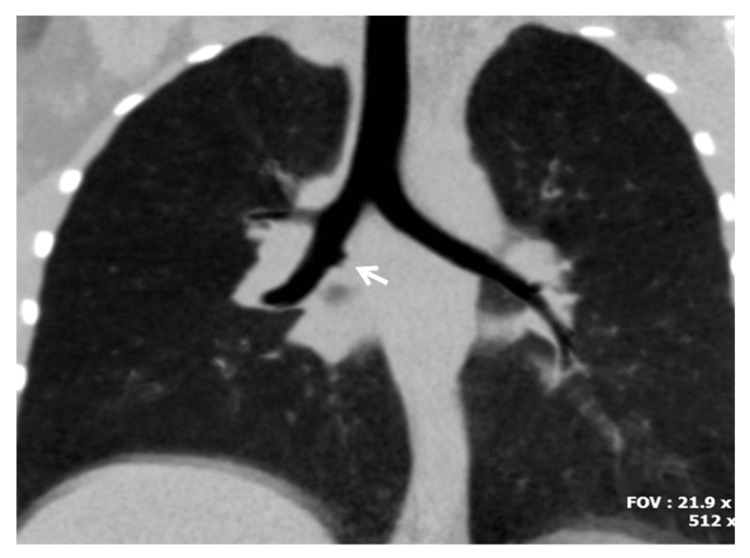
Computed tomography scan of accessory cardiac bronchus. Coronal reconstruction using minimum-intensity projection depicts a short cardiac bronchus (arrow). Image credit: Dr. Spyridon Prountzos and Prof. Efthymia Alexopoulou.
